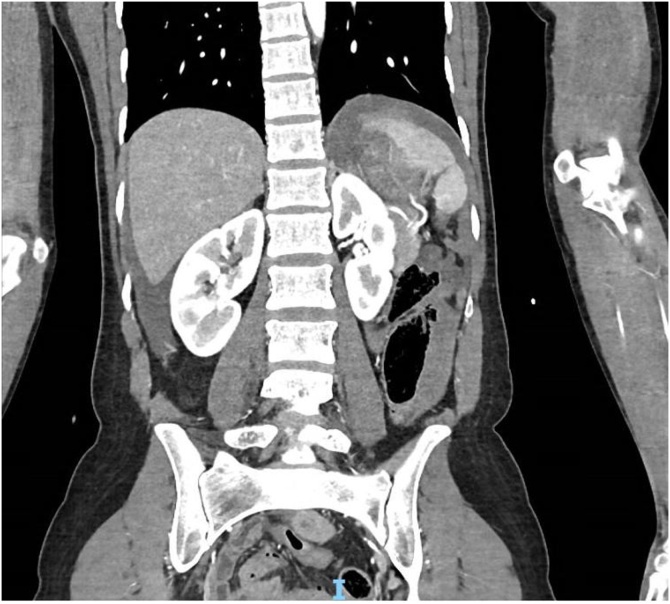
Coronal CT demonstrating splenic laceration with surrounding free fluid.
